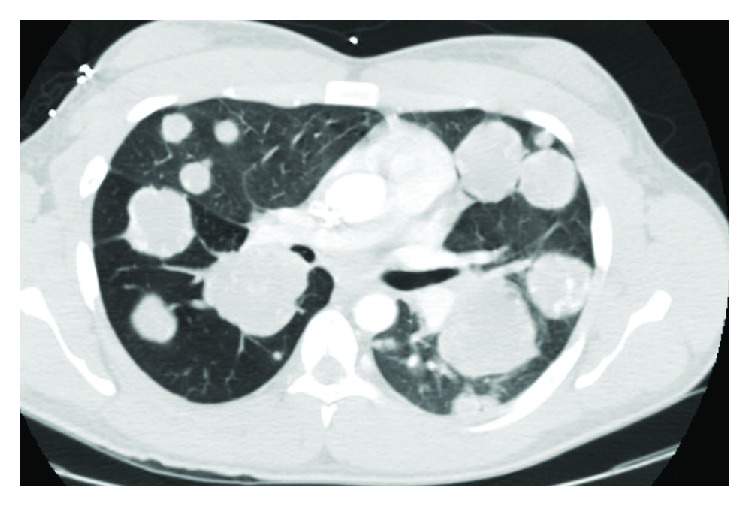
CT chest with pulmonary metastatic disease in a “cannonball” appearance.
